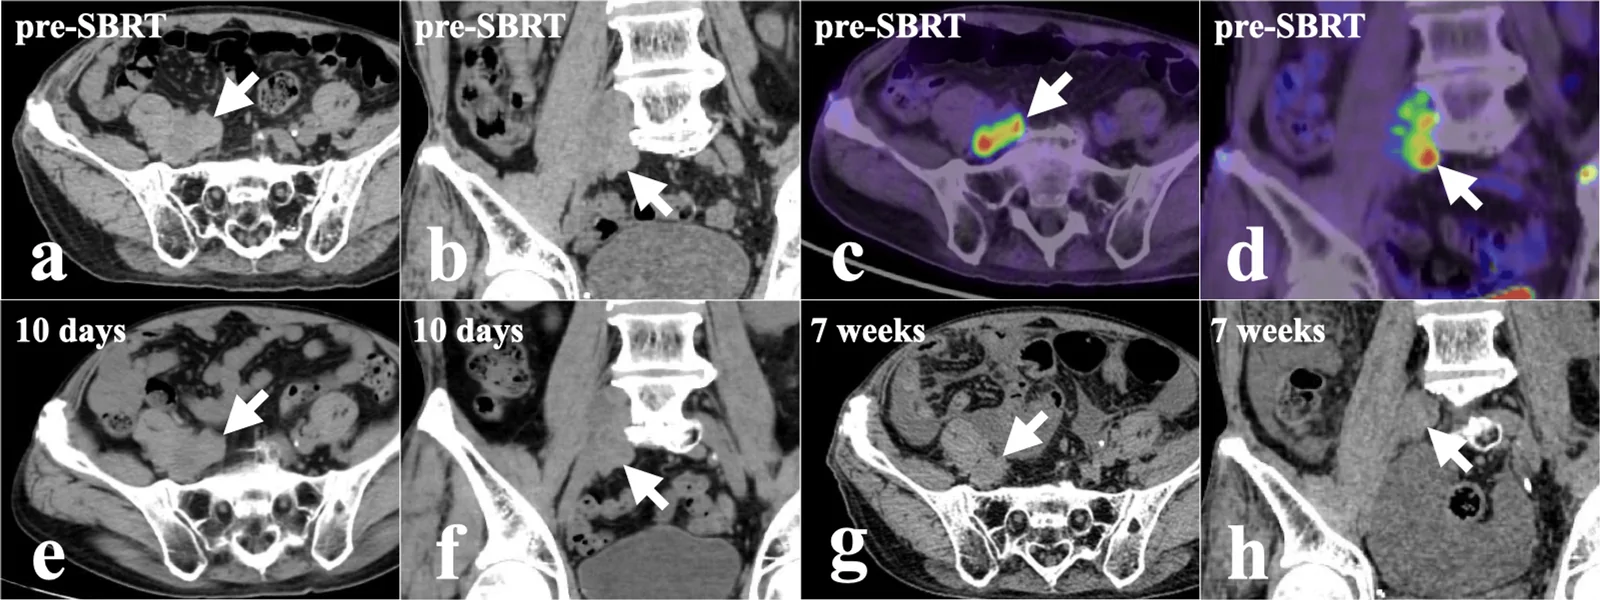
Metastatic lesion in the right abdomen (white arrows). (a-d) Images obtained before SBRT. (a, b) Non-contrast CT showing an isodense lesion. (c, d) PET/CT demonstrating intense FDG uptake in the lesion.  (e, f) Non-contrast CT obtained five days after SBRT initiation (the final day of treatment), showing tumor regression. (g, h) Non-contrast CT obtained seven weeks after SBRT, showing further tumor regression. (a, c, e, g) Axial views and (b, d, f, h) coronal views. SBRT, stereotactic body radiotherapy; CT, computed tomography; PET, positron emission tomography; FDG, 2-deoxy-2-[¹⁸F]fluoro-D-glucose.
